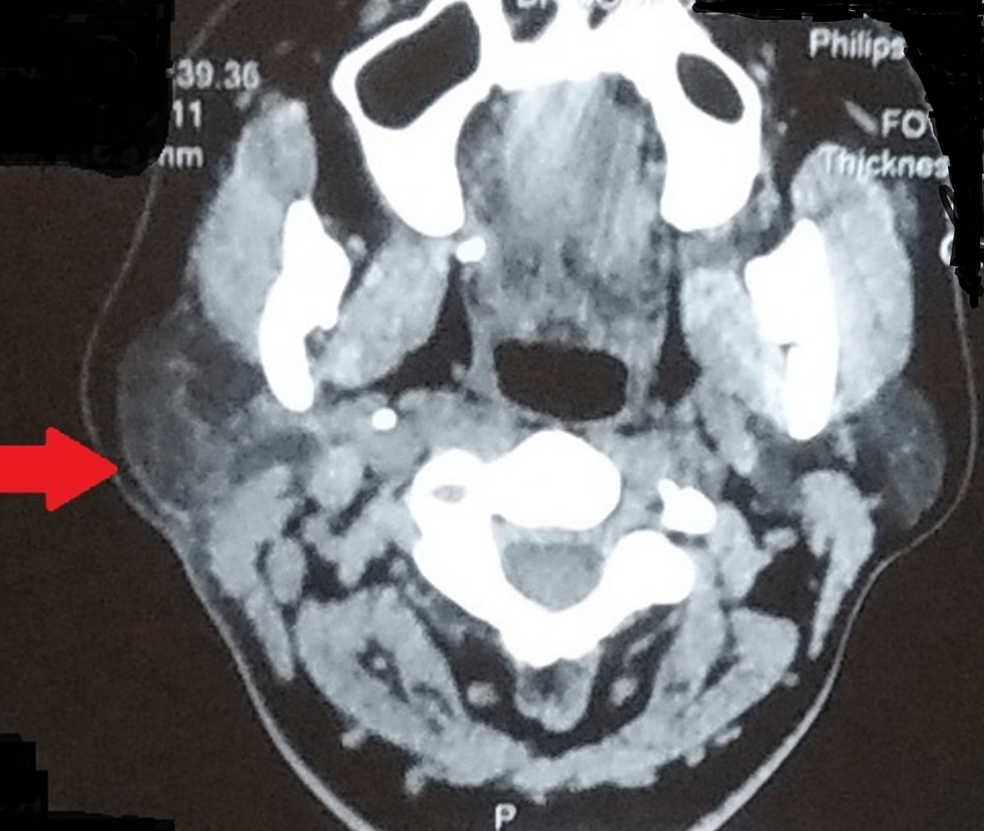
A multi-slice computed tomography (CT) of the neck (axial view) A multi-slice CT of the neck showing a small collection/abscess adjacent to the soft tissue density reaching up to the surface of the skin with a bulge
